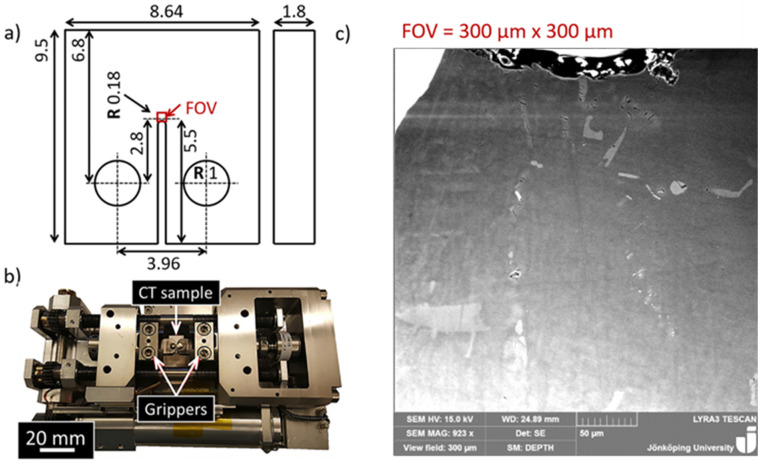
(a) Dimensions of the CT sample in mm [17]; (b) miniature stage for in situ cyclic tests [17]; (c) FOV of the A357 sample.
